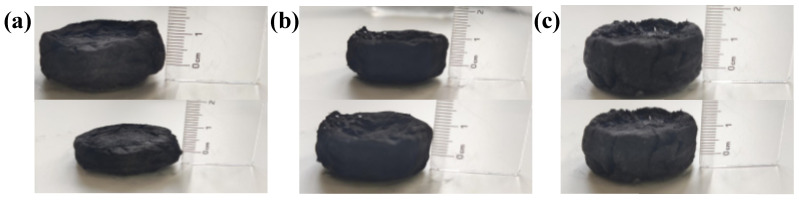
(a) CT-Fe, (b) CT-Fe-PVA and (c) CT-Fe-PVA-DM are aligned before and after extrusion at 1000 g.
